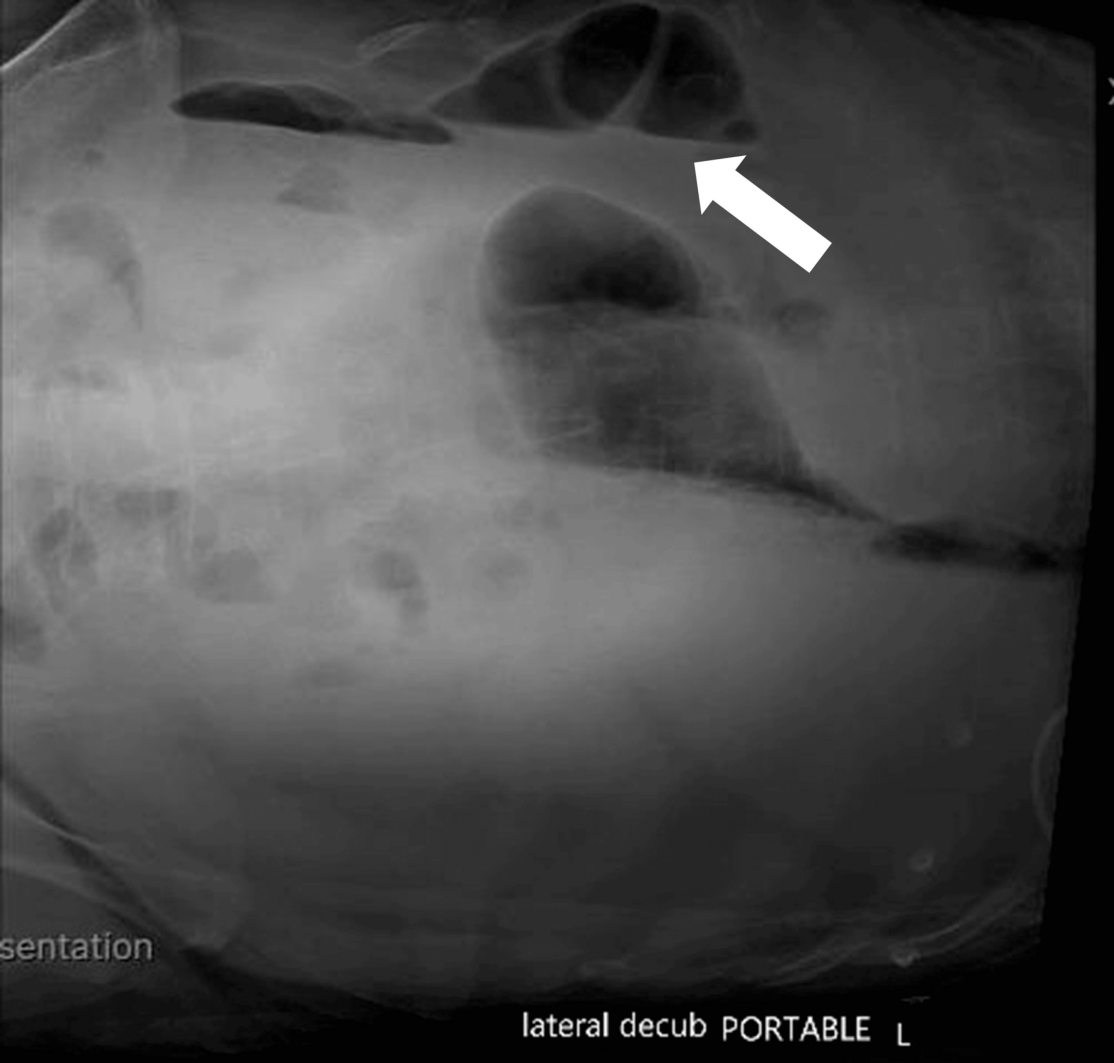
Abdominal radiograph demonstrating ileus with multiple air-fluid levels Abdominal radiograph series obtained on the day of presentation, including a lateral decubitus view, demonstrates diffuse gaseous bowel distension with multiple air-fluid levels, consistent with ileus
